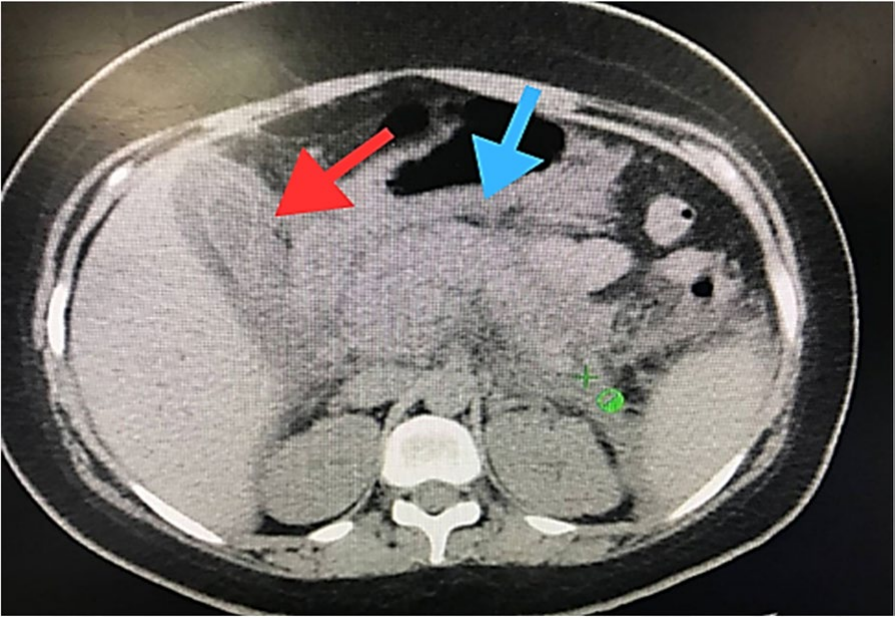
Coronal CT scan of the abdomen showing biliary sludge, gallstones (indicated by the red arrow), and evidence of pancreatitis (indicated by the blue arrow)
